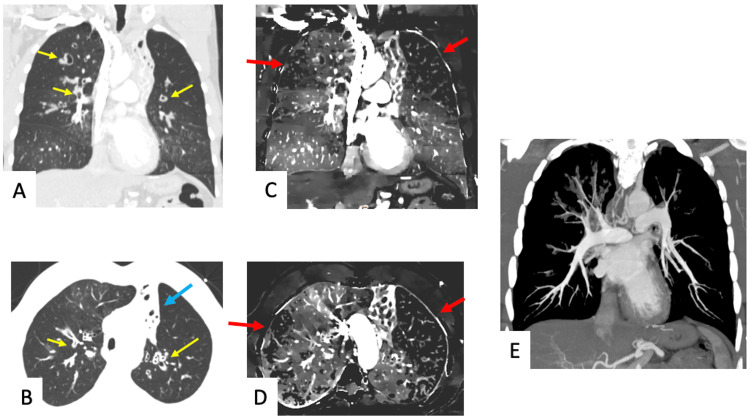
A 42-year-old male with history of chronic cystic fibrosis presenting with acute onset hemoptysis. Coronal and axial CT images in lung window setting (A, B) shows extensive upper lobe predominant bronchiectatic changes and multifocal mucous plugging (yellow arrows). Complete left upper lobe lung collapse is also visualized (blue arrow). The corresponding coronal and axial iodine density maps (C, D) demonstrate multiple wedge-shaped defects particularly in the left lung apex (red arrows), likely from hypoxic vasoconstriction given extensive mucous plugging and no pulmonary embolism as demonstrated by maximum intensity projection CT image (E). CT: Computed tomography
